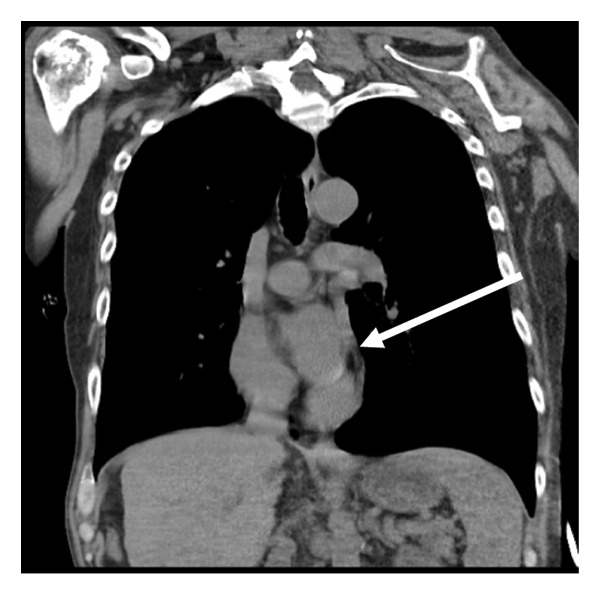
Previous (2019) coronal CT showing the left hilar PLSVC path (white arrow) coursing towards the coronary sinus.
